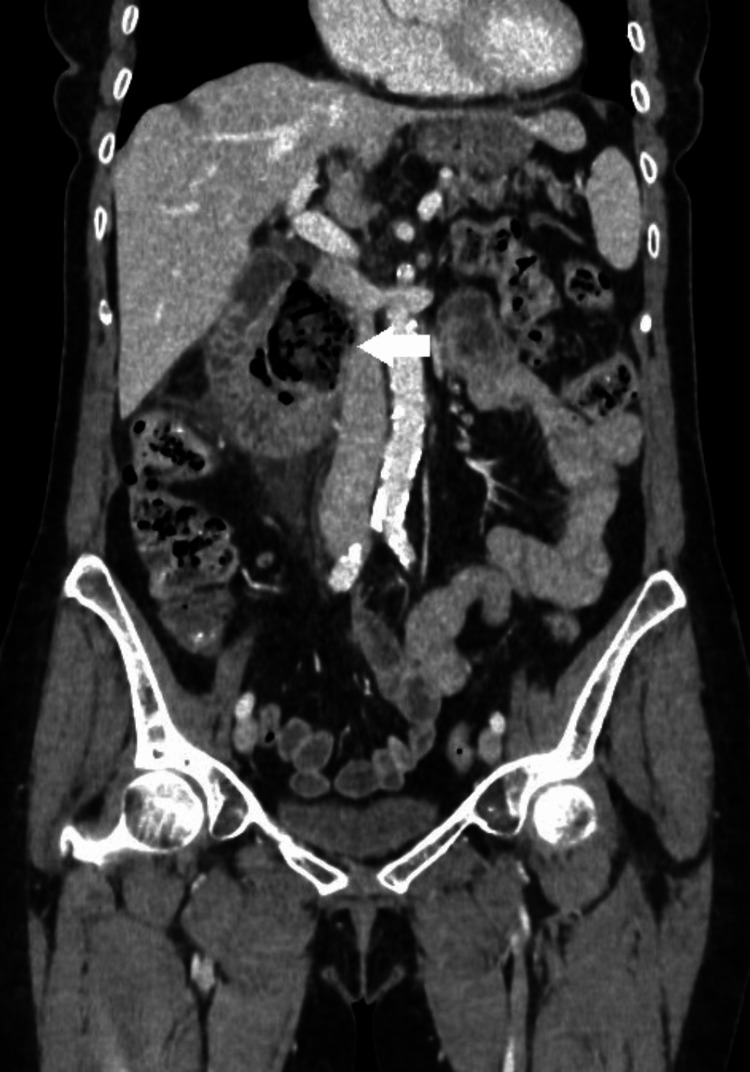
Initial CT of the abdomen and pelvis - coronal reconstruction. Duodenal diverticulum (white arrow) with duodenal wall edema, peridiverticular "fat-stranding," and extraluminal air bubbles - CT signs of perforation.
